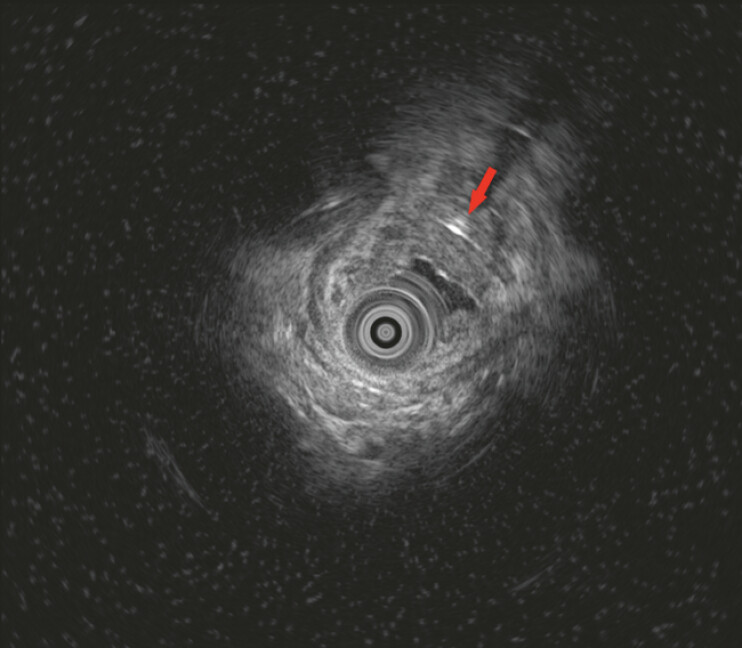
Endoscopic ultrasound (EUS) revealed punctate and linear hyperechoic foci.
